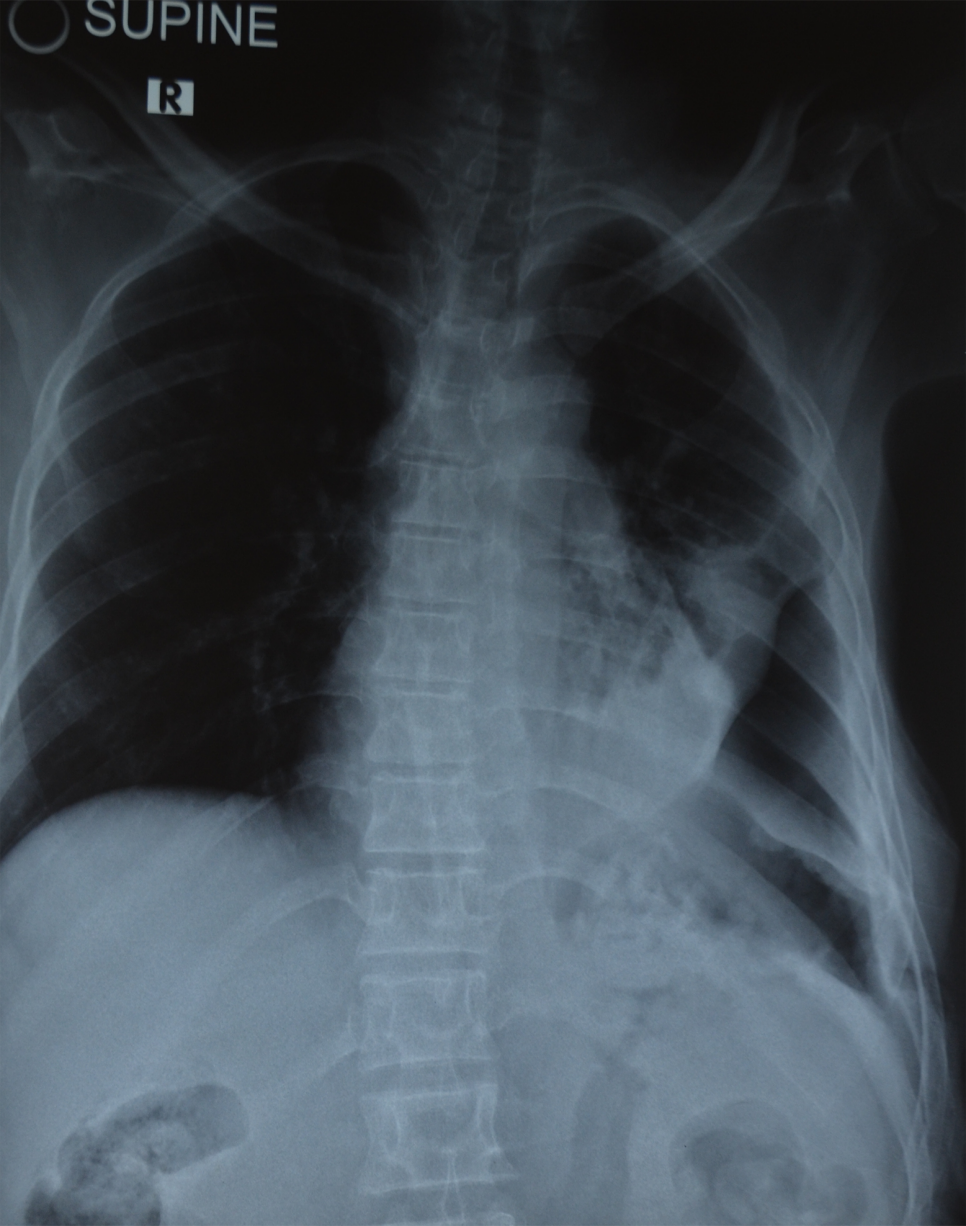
Chest X-ray showing soft tissue in left lateral part of the chest wall, left lower lung mass collapse, decrease lung mass with left-sided pneumothorax.
